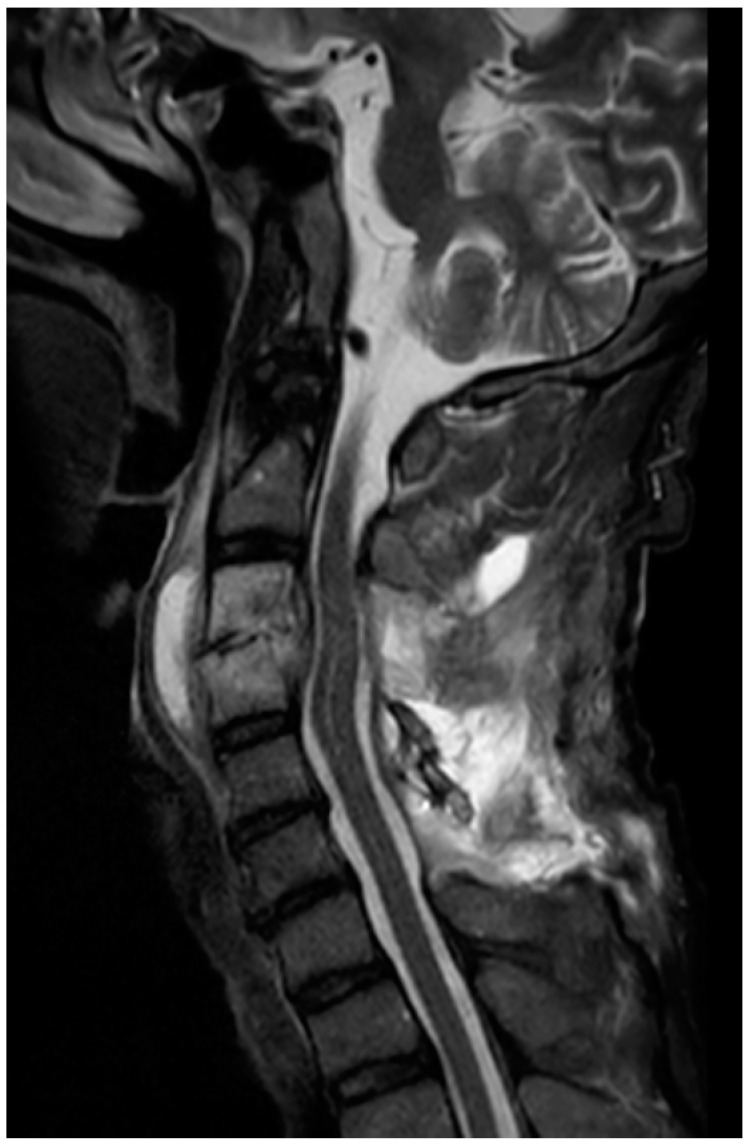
MRI T2 STIR on day 23. Although anterior compression at the C3–4 level has progressed, spinal canal stenosis has not developed because laminectomy had been performed.
